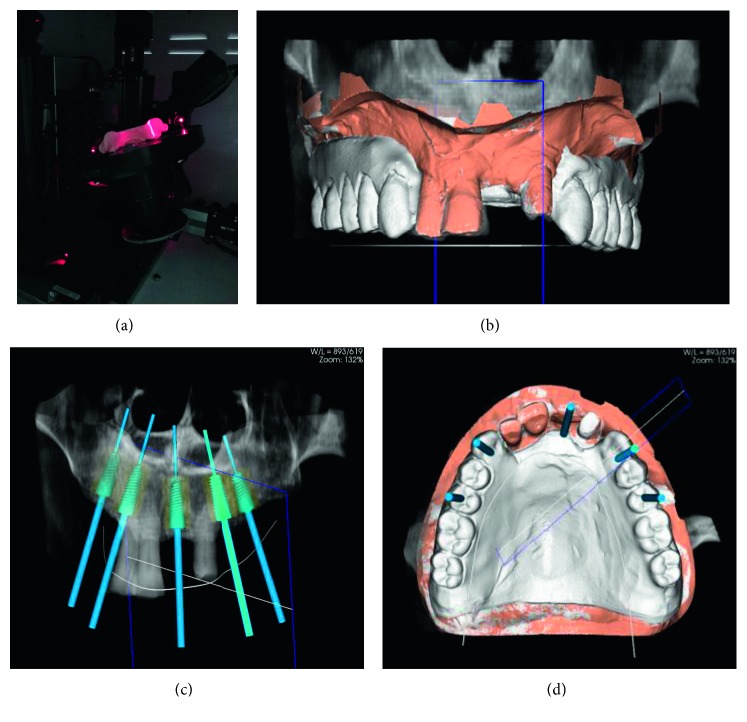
(a) Stone model scanning; (b) coupling DICOM files from CBCT with STL files from model scan; (c, d) computer planning of implant position.
